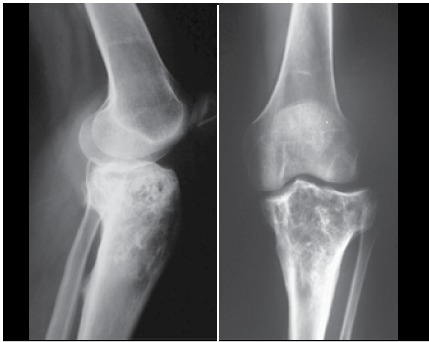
X-ray image of a primary bone lymphoma in the proximal tibia (after chemotherapy).
